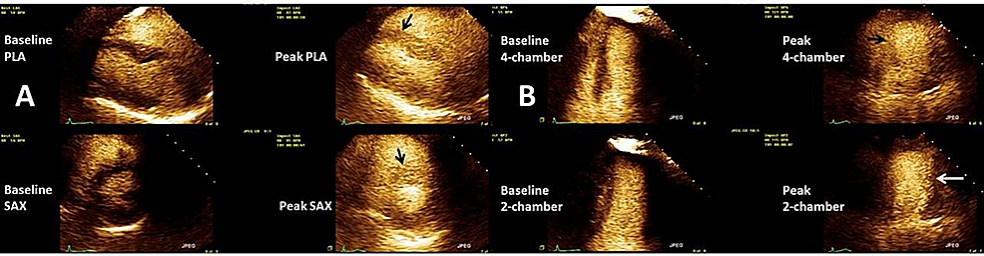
Stress echocardiogram from clinical case 2 (A) Standard parasternal long- and short-axis views of the left ventricle both at rest and post-exercise using a treadmill stress echo protocol. Please note that the black arrows demonstrate the lack of contractility of the anteroseptal and anterior walls at peak exercise. (B) Standard four- and two-chamber apical views of the left ventricle showing the same test phases as in the previous. Please note that the black arrow demonstrates the lack of contractility of the distal inferoseptal wall. The white arrow shows anterior wall ischemia at peak exercise
